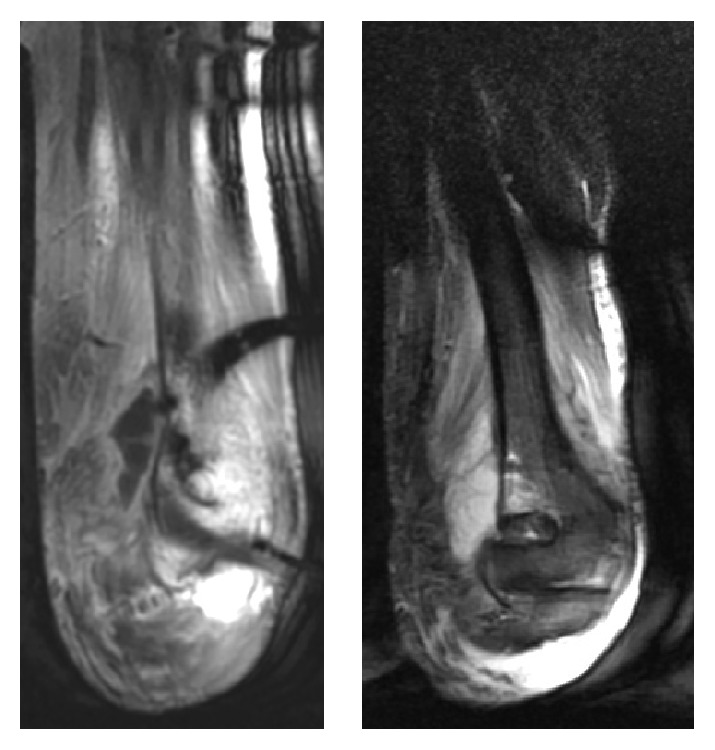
MRI (coronal T1 and STIR) showing a collection of 51 × 23 mm with bone edema in the humerus supratrochlear region with apparent cortical integrity.
